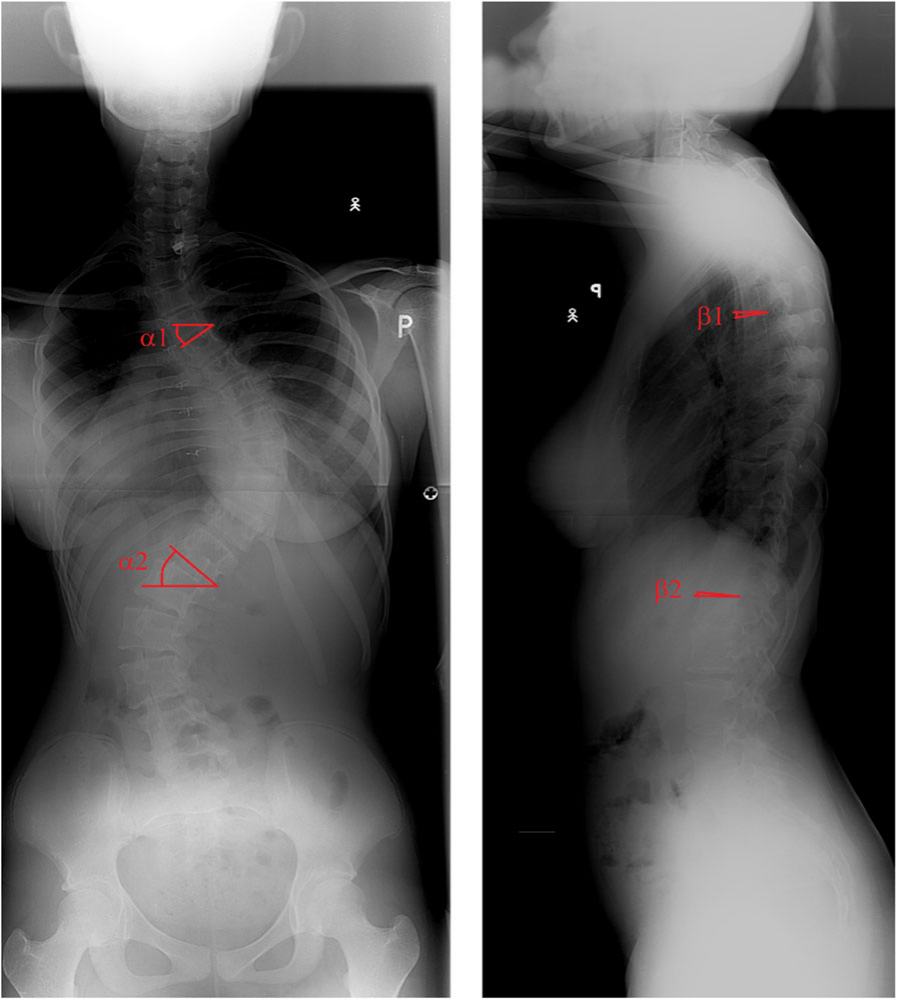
Four angle method for evaluating the angle between the upper and lower endplates of the scoliosis curve based on two X-rays scans: posterior-anterior and lateral
